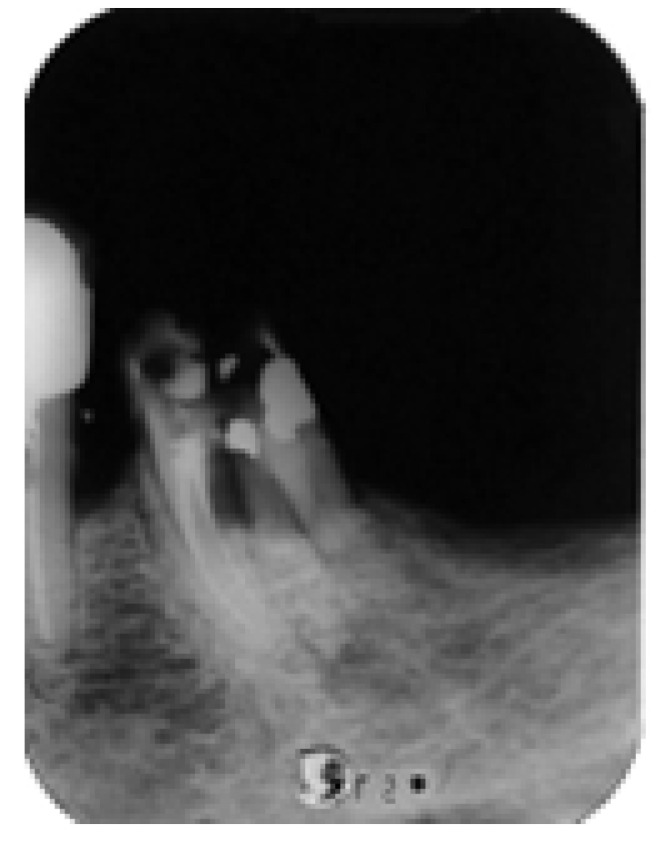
X-ray after crown removal showing the existence of the second distal root of tooth #36.
